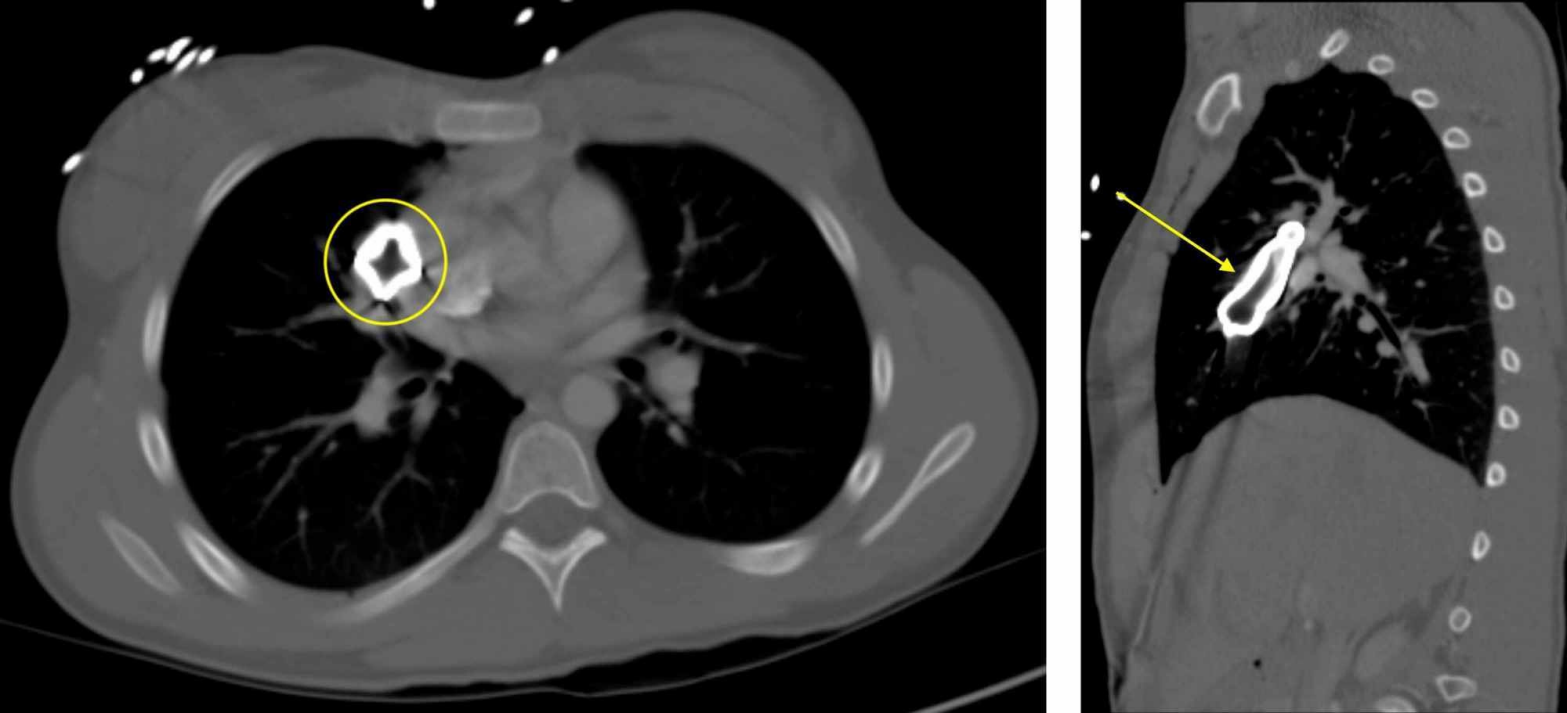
Axial and sagittal CT chest with intravenous contrast display a large retained metallic foreign body (yellow) that penetrates the right chest wall in an anterior to posterior trajectory and extends superiorly through the mediastinum and right hemithorax abutting the adjacent right heart border, hilum, and vasculature
